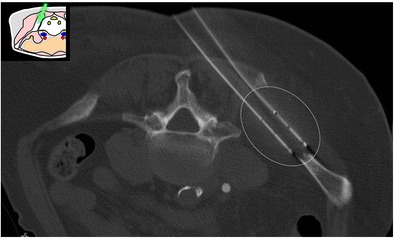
A 65-year-old woman with lung cancer and painful iliac metastasis. Axial CT shows the cryoablation probes inserted along the greater axis of the iliac wing and the hypodense ice ball covering the lesion (*dotted line*)
